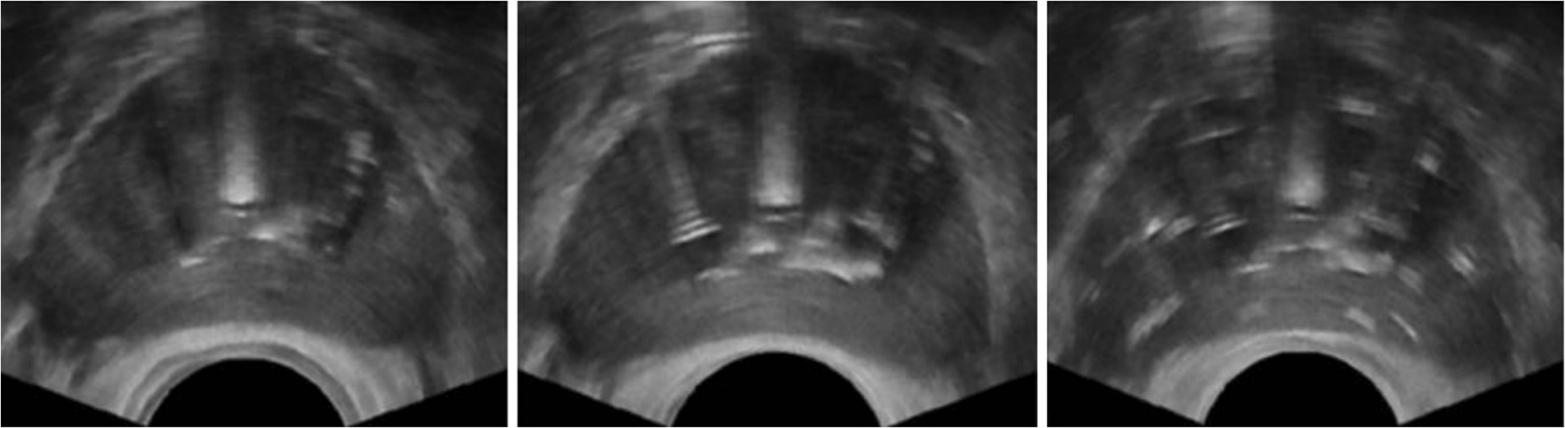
Example of TRUS (transrectal ultrasound) image acquisition of the prostate (mid-gland) at 3 different time points during the course of the implant (all in the operating room). Left image corresponds to baseline volume before the implant (V1), middle image to prostate volume after the insertion of 2 fixation needles (V2) and right image to prostate after the completion of the implant (V3).
